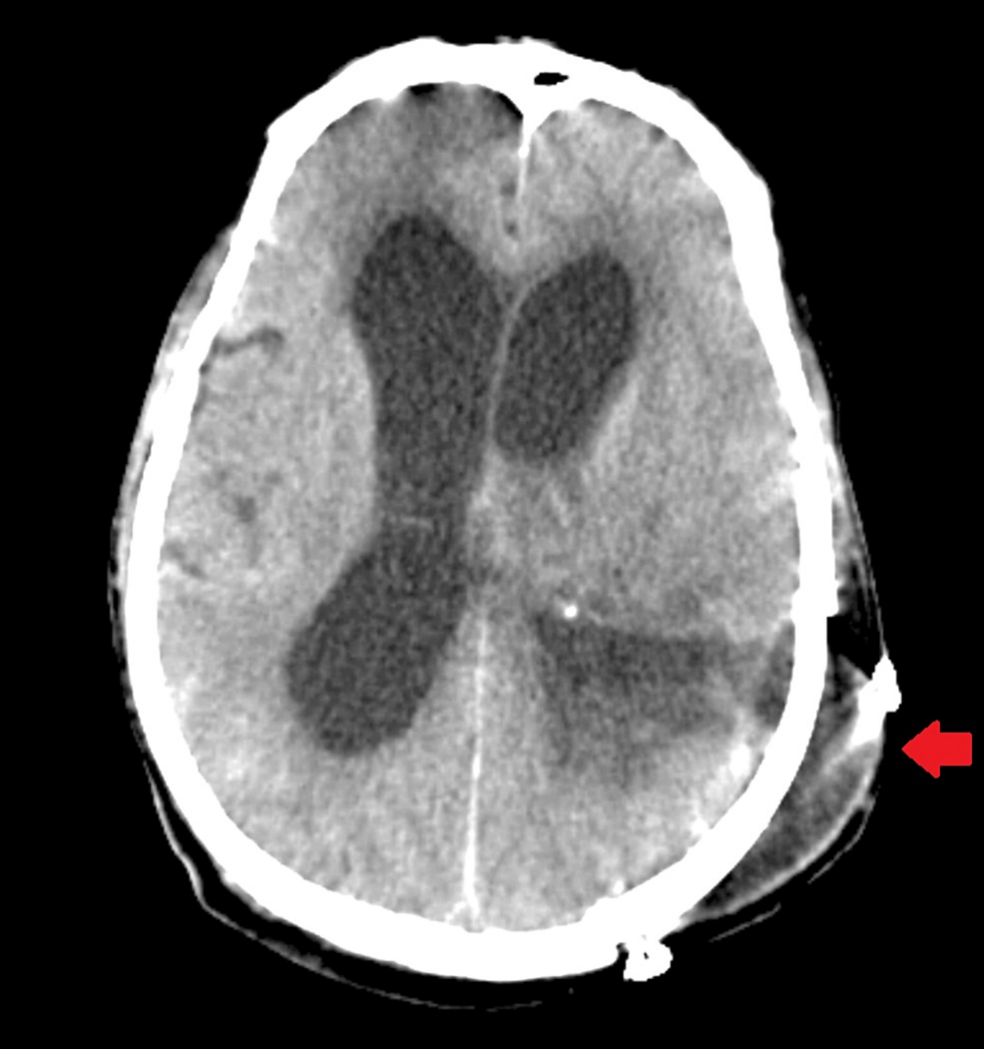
The head CT of the patient revealed bilateral dilatation and communicating hydrocephalus, along with evidence of a brain tumor.
